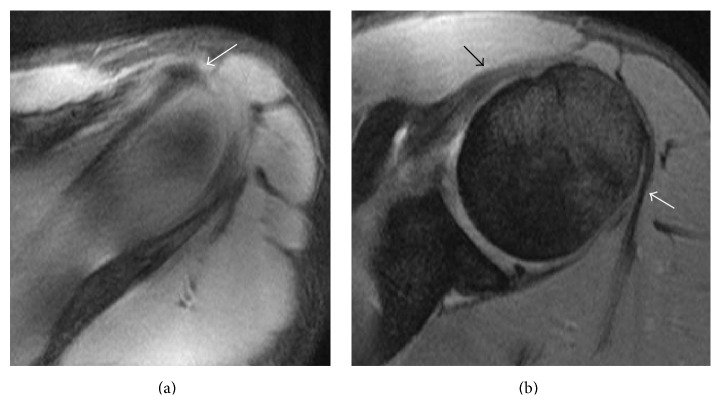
Axial DP FS SE MR images of the left shoulder (TR = 475; TE = 13.4; NEX = 2; EC = 1). (a) The supraspinatus tendon is visible, without any discontinuity (white arrow). (b) The subscapularis tendon (black arrow) and the infraspinatus tendon (white arrow) show normal thickness and hypointensity, without tears.
